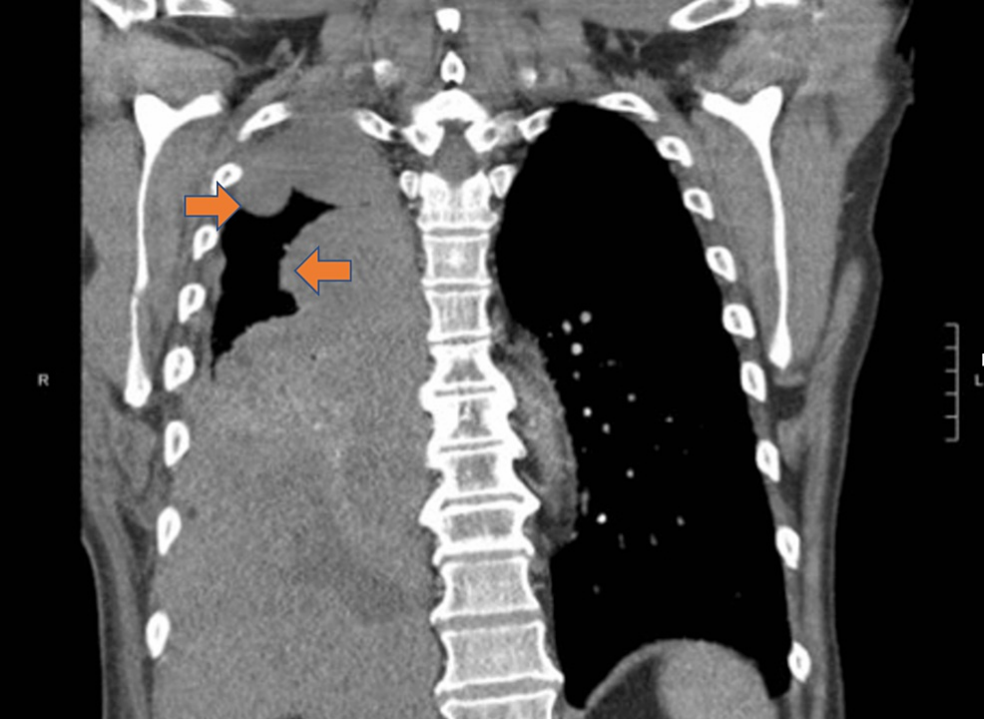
Chest CT with intravenous contrast on readmission two weeks after initial discharge. Extensive enlargement of right pleural masses (orange arrows), consistent with progression of pleural malignancy.
